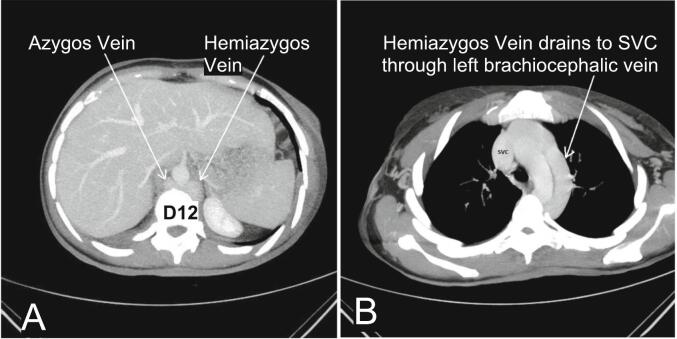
Axial maximum intensity projection (MIP) images of contrast-enhanced CT (portal phase) demonstrate the convergence of the azygos-hemiazygos system at the level of the D12 vertebra (A). Subsequently, the hemiazygos vein drains into the SVC through the left brachiocephalic vein (B).
